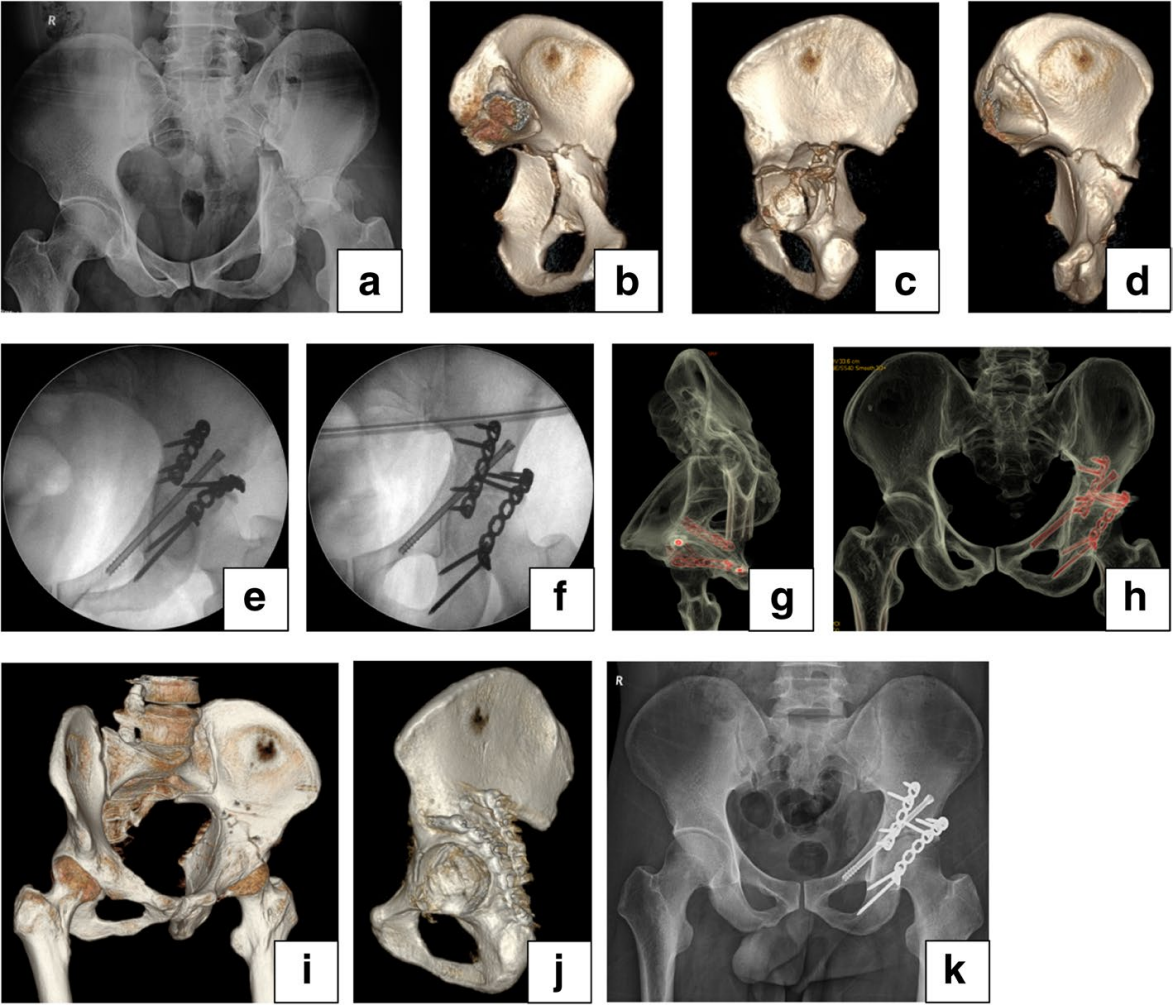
Male, 39-year-old, car accident, a T-shaped acetabular fracture associated with posterior wall fracture, treated with the K-L approach combined with percutaneous anterior column screw fixation under robotic guidance. a Preoperative X-ray antero-posterior image shows an acetabular fracture associated with femoral head dislocation. b-d 3D CT images of a acetabula show a T-shaped acetabular fracture associated with the communicated posterior wall. e, f Intraoperative images reveal anatomic reduction of the acetabular fracture. g A postoperative axial image of the anterior column demonstrates the screw is located at the bony corridor. h-g, 3D CT images show anatomic reduction of the acetabular fracture. k The antero-posterior X-ray image three months after surgery shows fracture healing
